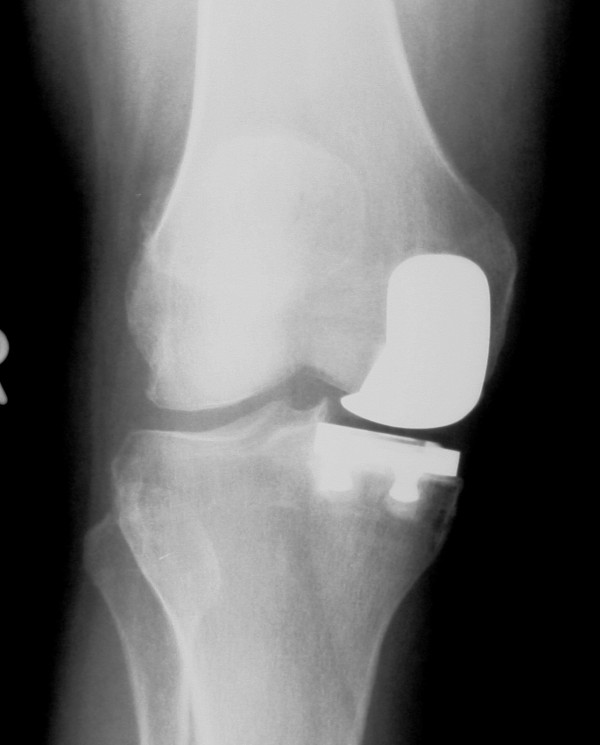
Post-operative antero-posterior radiograph of the same patient shown in Figure 1 at 6 week follow-up visit.
